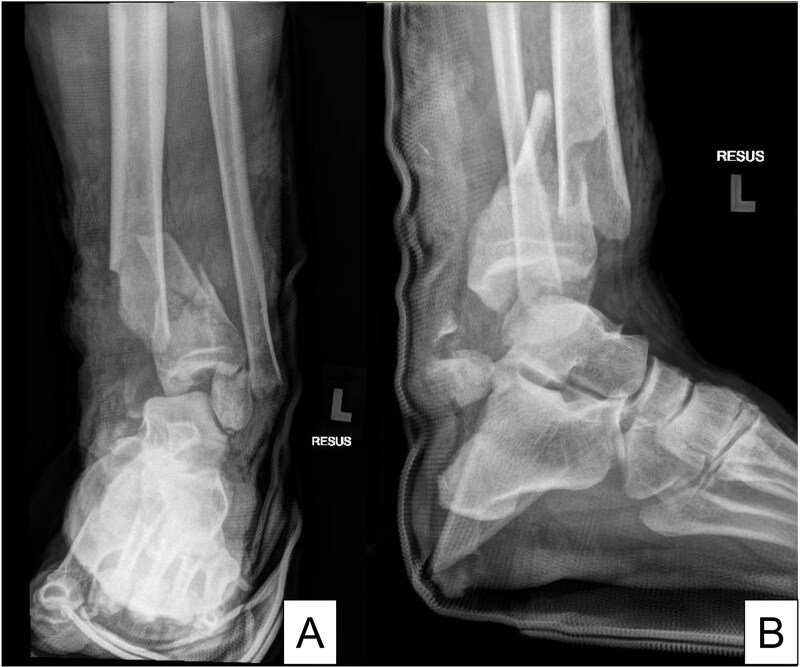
X-rays of the left ankle. (A) Anteroposterior and (B) lateral views.
